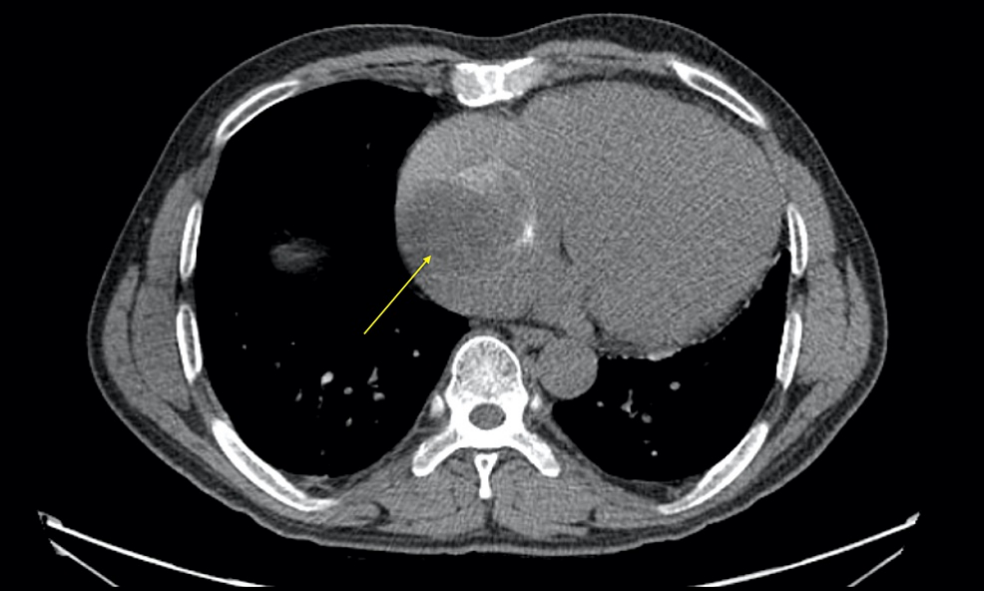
Transverse view of CT of chest Transverse view of CT of chest showing a mass in the cardiac area with calcifications (yellow arrow).
